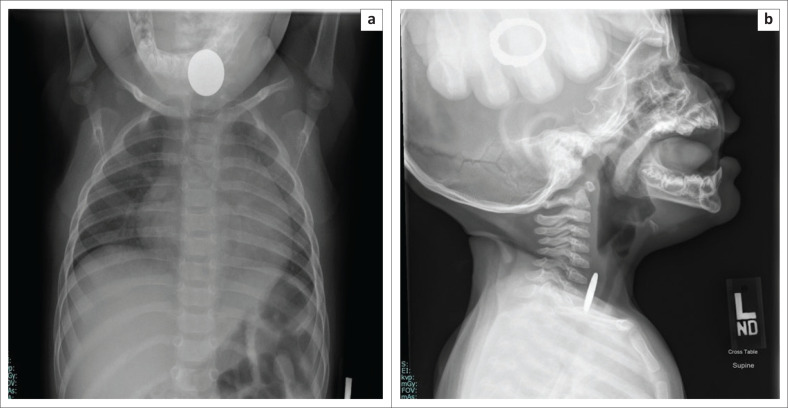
Frontal (a) and lateral (b) radiographs of the neck reveal a swallowed coin in the proximal oesophagus of a 10-month-old child who presented with respiratory distress and drooling.
